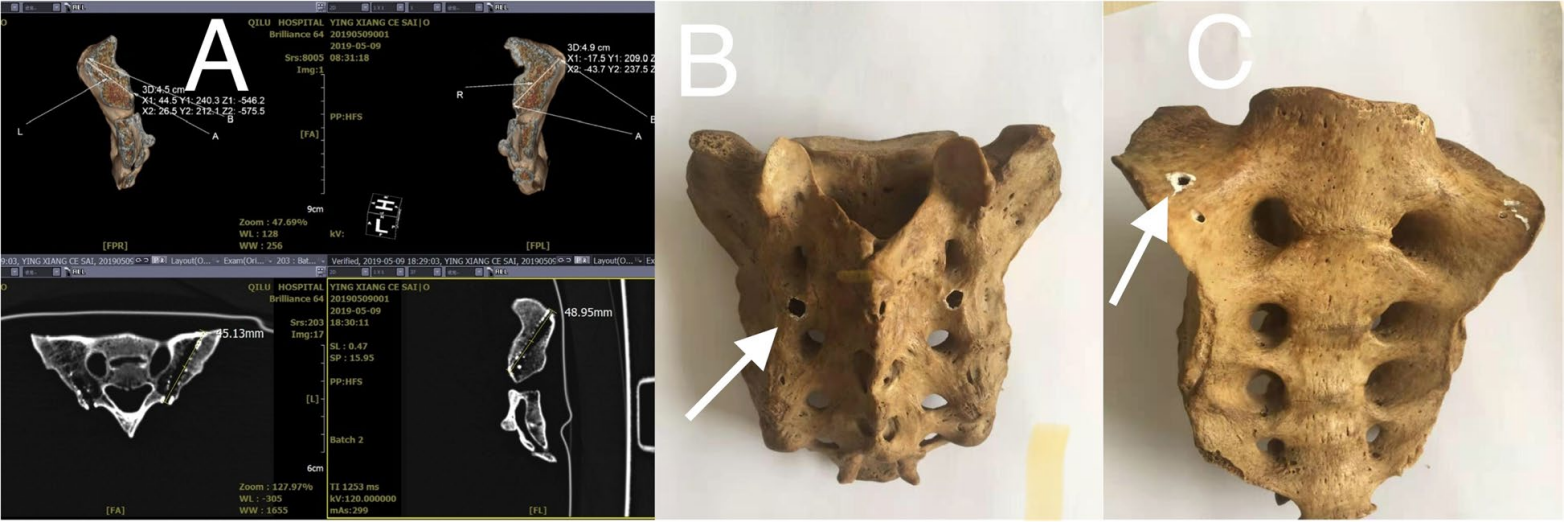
Imitation by CT and anatomical measurements of the screw trajectory
